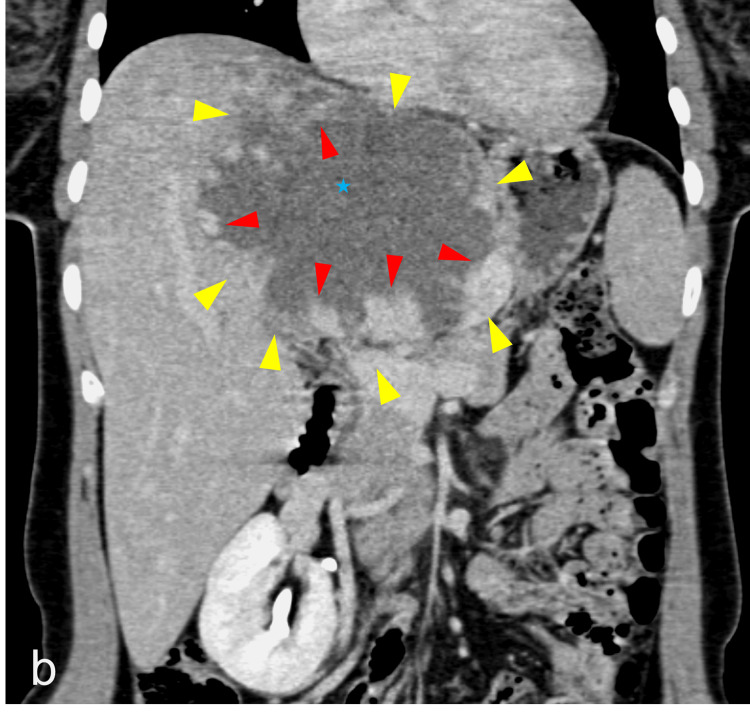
Coronal image of intravenous contrast-enhanced CT of the upper abdomen demonstrating a giant cavernous hemangioma occupying the left lateral segment of the liver, with interrupted nodular peripheral enhancement (red arrowheads) and a small central scar (blue star). The lesion margins are delineated by yellow arrowheads.
